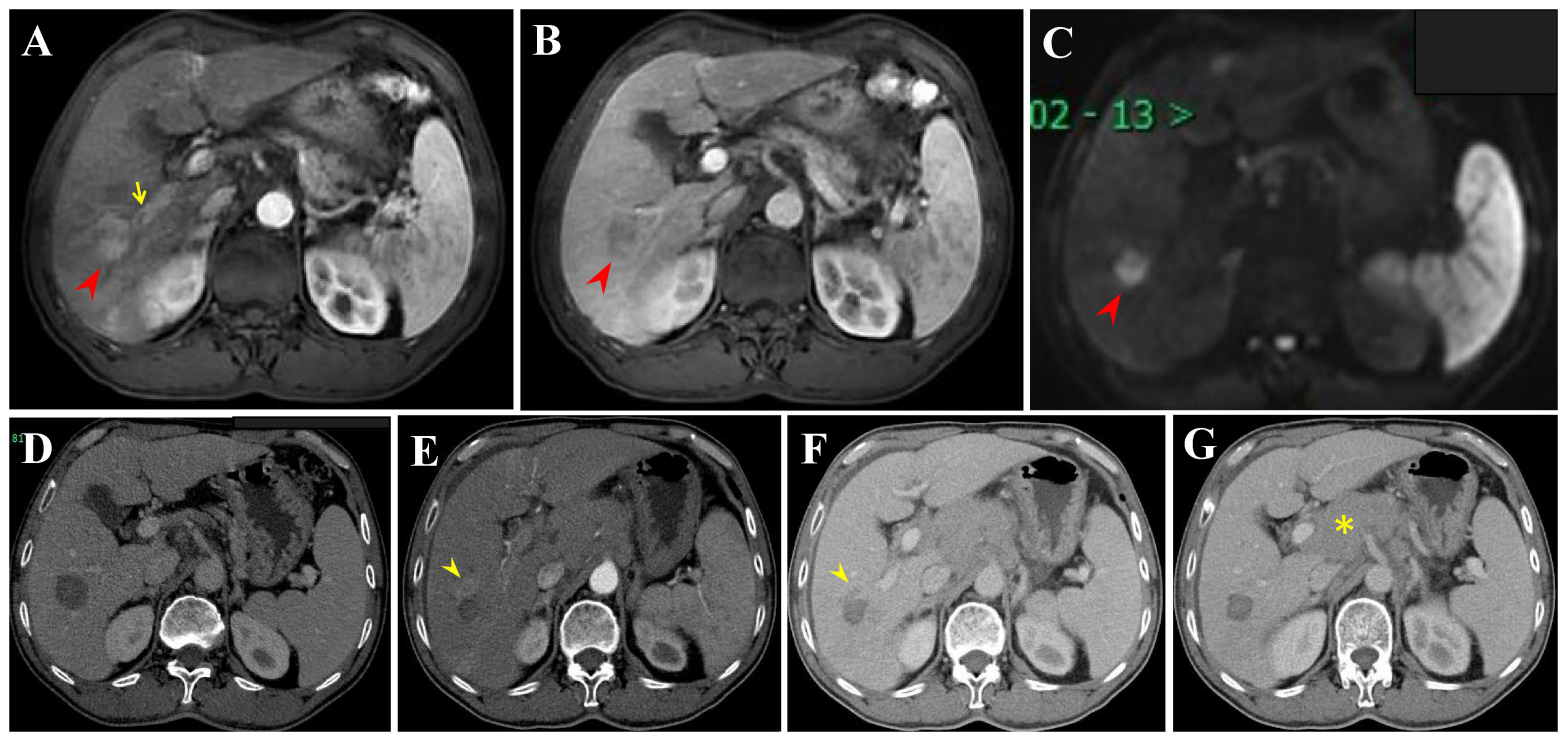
Extrahepatic recurrence (ER) and LTP after RFA alone for perivascular HCC in a 57-year-old woman. (A-C) DCE-MRI scans show washin at hepatic arterial phase and washout at portal venous phase (arrowheads). (D) CECT scan obtained during portal venous phase 1 month after RFA showing the complete ablation zone adjacent to the portal vein. (E, F) CECT scans obtained during hepatic arterial phase and portal venous phase 19 months after RFA showing the LTP, a small arterial enhancing nodule (arrowheads), with washout at portal venous phase. (G) CECT scan obtained during hepatic arterial phase (not shown) and portal venous phase 19 months after RFA showing the ER (asterisk). Then, the patient received locoregional theraphy (TACE + RFA) plus systemic treatment (not shown).
